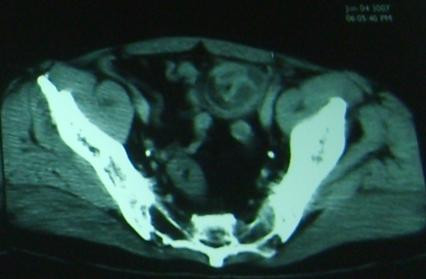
A computed tomography scan showing an image (arrow) in the left iliac fossa that suggests an intussusception.
